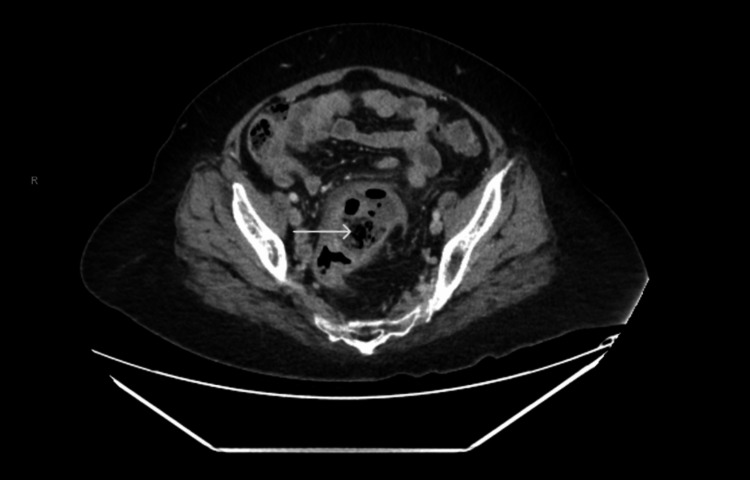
CT abdomen and pelvis (axial view) CT of the abdomen and pelvis (axial) demonstrating sigmoid GCD (arrow) with associated surrounding inflammation.
